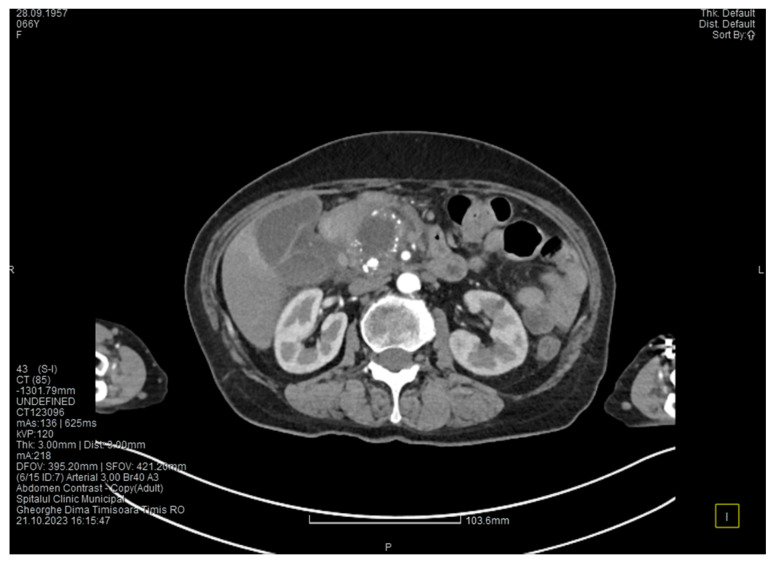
CT scan at the fourth episode of acute pancreatitis shows a slightly increased cyst and calcifications in the pancreatic head.
